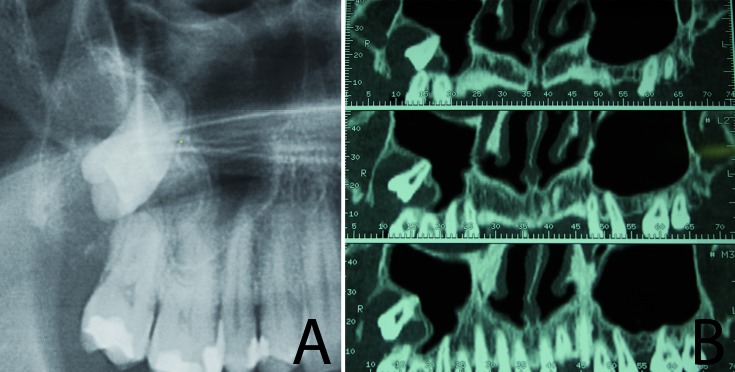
a.Panoramic radiograph showing a large radiolucency in close proximity to the third molar; b. Computed Tomography showing a radiolucent area around the third molar and comprising the second molar.
